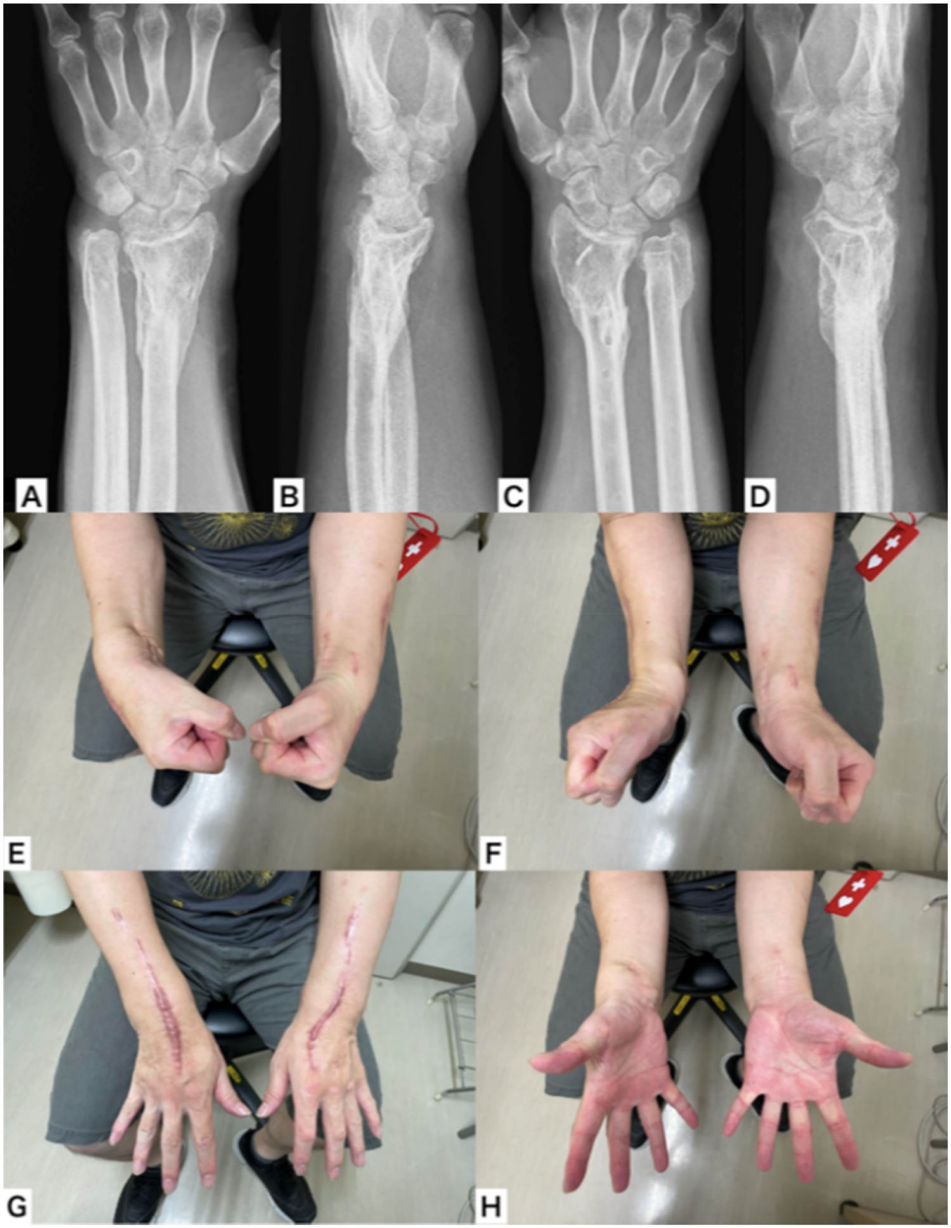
Plain x-ray, appearance, and range of motion of both wrists 1 year postoperatively. (A,B) Plain x-ray of the left wrist; (C,D) plain x-ray of the right wrist; (E) active palmar flexion; (F) active dorsiflexion; (G) forearm pronation; (H) forearm supination.
